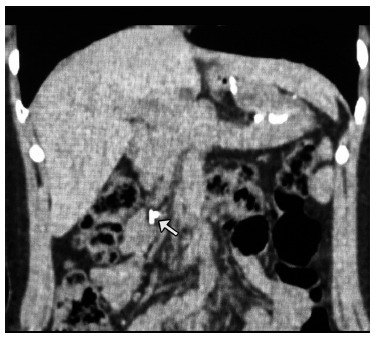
Abdominal CT interpreted as normal. However, subsequent surgical review revealed clips to the right of the spine, signaling Petersen’s hernia
